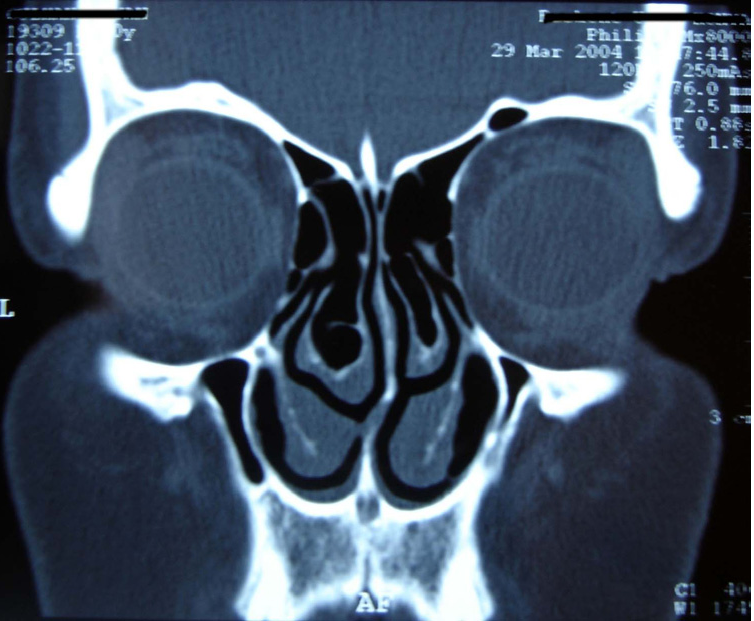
CT scan shows septal deviation and bilateral concha bullosa.
